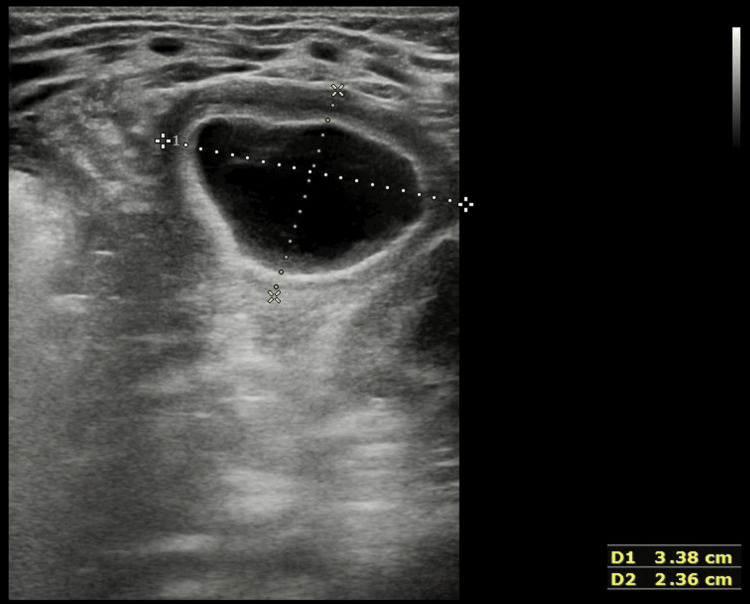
Abdominal ultrasound demonstrating a cystic lesion with a layered (stratified) wall located in the left epigastric region.
